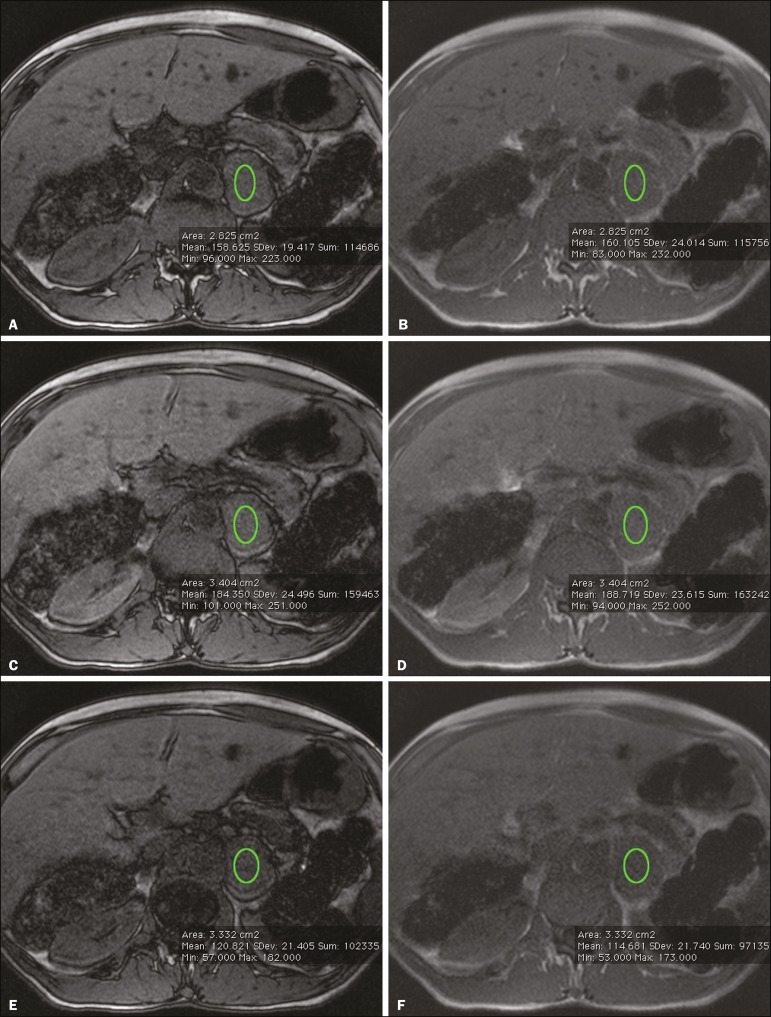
Chemical shift MRI. Placement of the ROI to cover one half to two thirds of the adrenal mass: axial out-of-phase (A,C,E) and in-phase (B,D,F) sequences.
